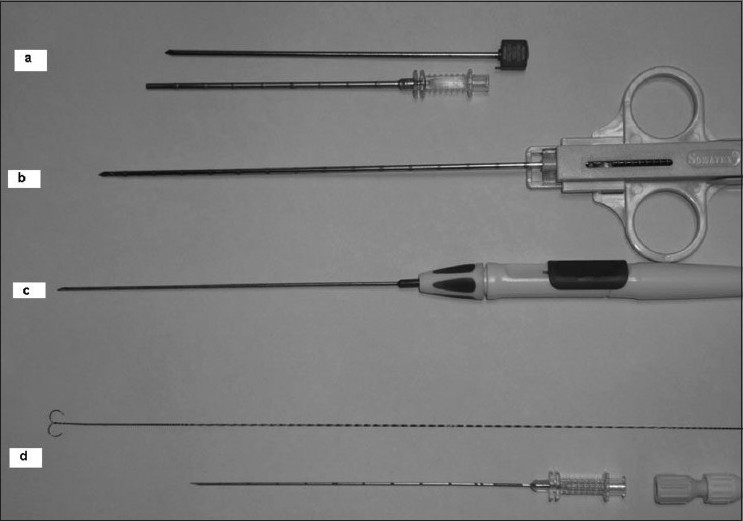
Instruments. MRI-compatible 13-G coaxial needle with trocar and cannula (a), MRI-compatible 14-G biopsy gun (b), MRI-compatible marker needle (c), MRI-compatible hook wire, cannula and locking device (d)
